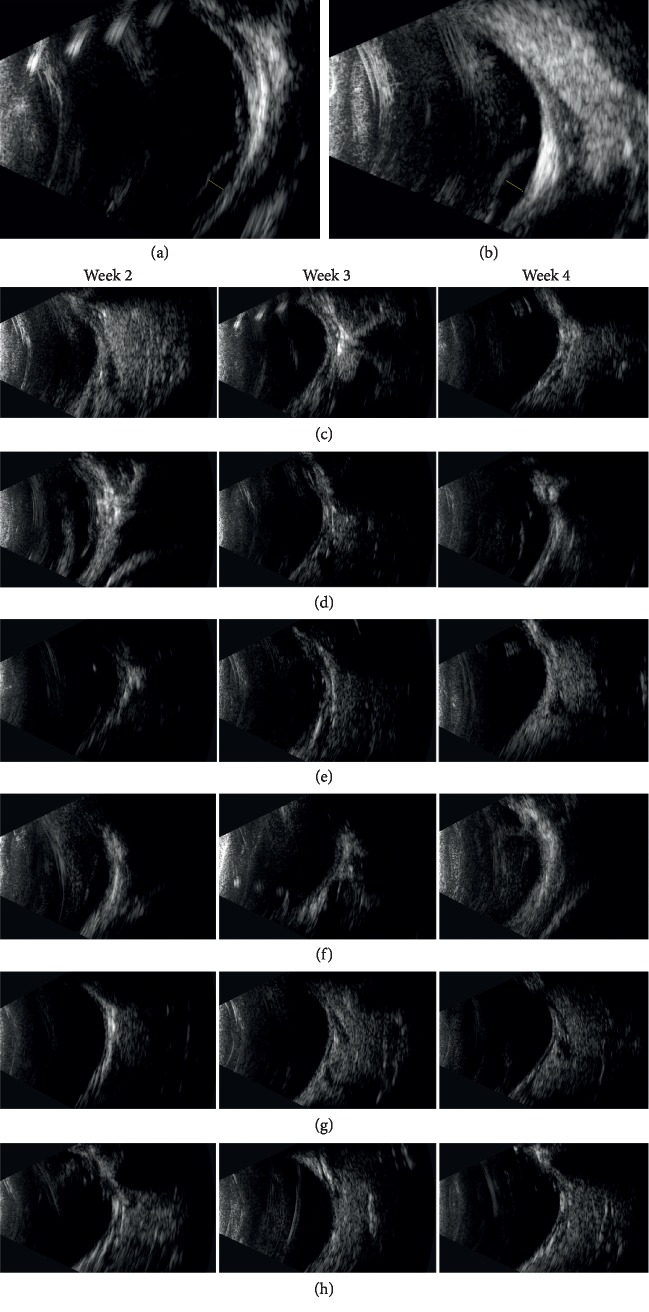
(a, b) A slight uplift of the retina caused by the volume of the drug solution was observed in two eyes (two rabbits) of the subretinal group 24 hours after injection. The height of the uplift was measured (the yellow straight line). (c–h) B-ultrasonography of the six eyes (six rabbits) in the subretinal group 2 to 4 weeks after conbercept injection. The slight uplift of the retina reverted to a flat state in (e) and (f). No retinal detachment or vitreous hemorrhage was observed.
